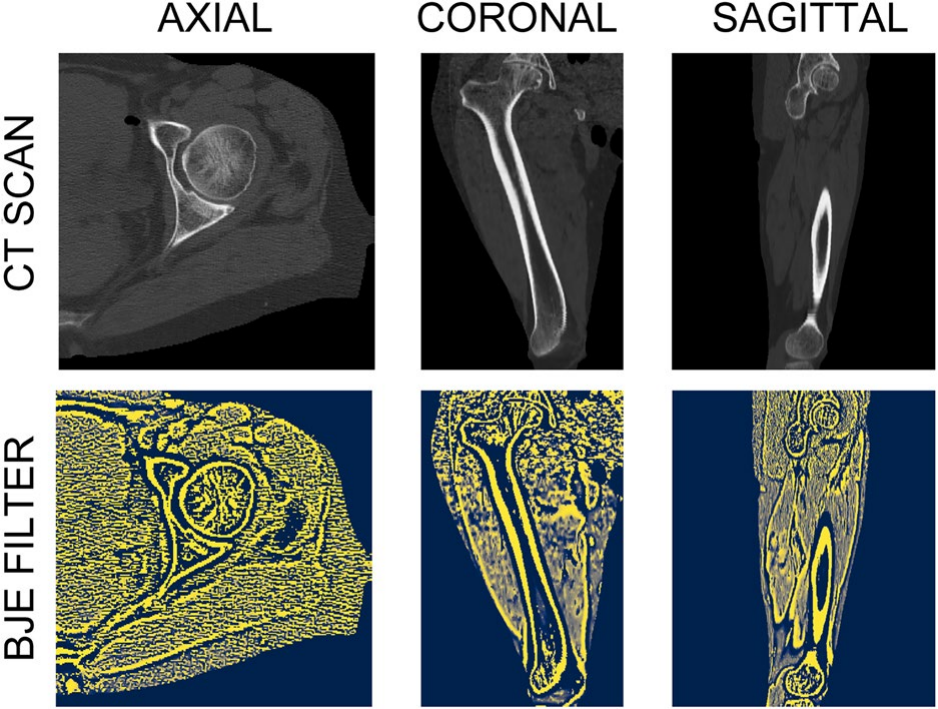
BJE filter: CT scans before (upper panel) and after (lower panel) its application. The upper panel shows the original CT image in HU, while the lower panel displays the Boneness map, which is obtained by applying the BJE filter. This filter generates a range of values from − 1 to 1, with − 1 representing the joints and 1 representing the cortical bones. The Boneness map highlights the joint and cortical areas more prominently than what is seen in the original scan.
